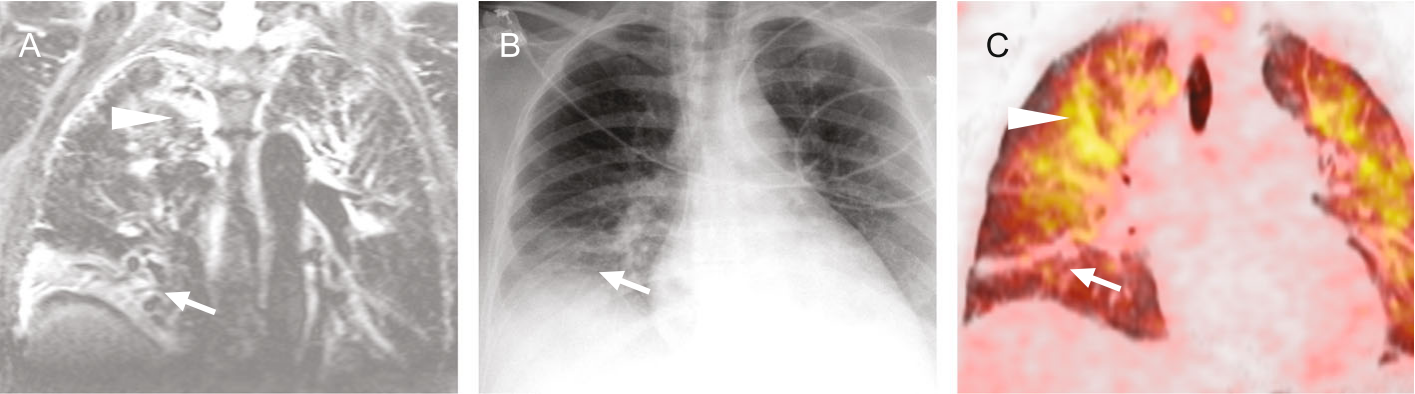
56-year-old patient with pulmonary infiltrations as infectious focus. Juxtaposition of coronal T2-STIR a, conventional radiography of the chest b and coronal PET-CT c in chronological order. One day elapsed between each image. Note that the middle lobe atelectasis (arrow) resolves over time, while the paramediastinal infiltrations (arrow head) increase. The conventional radiography c shows signs of the atelectasis in the right lower zone but no clear sign of the paramediastinal pulmonary infiltration
